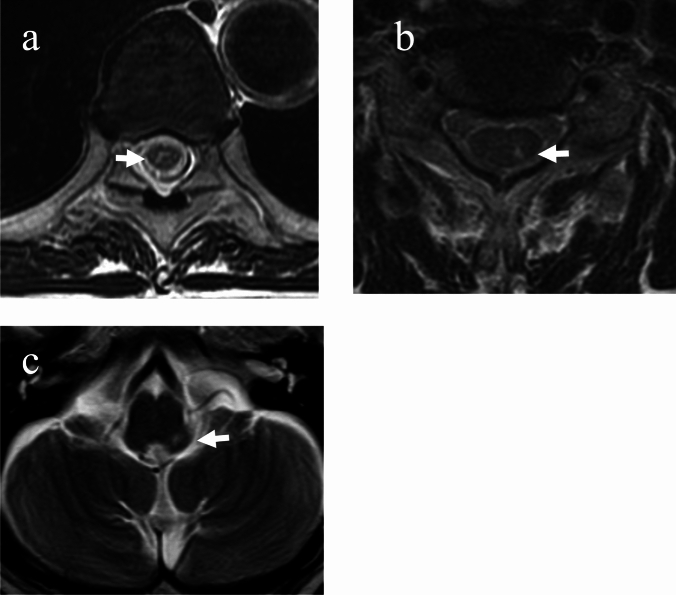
T2 HIZ of spinal cord dorsal horn in spinal MRI. Panels a, b, and c show examples of the T2 HIZ( +). White arrows indicate the lesions. a Thoracic spinal MRI of a patient with herpes zoster in the right T9 area. b Cervical spinal MRI of a patient with herpes zoster in the left C8. c Head MRI of a patient with herpes zoster in the left third trigeminal area. *T2 HIZ* T2 high-signal-intensity zone; *MRI* magnetic resonance imaging
